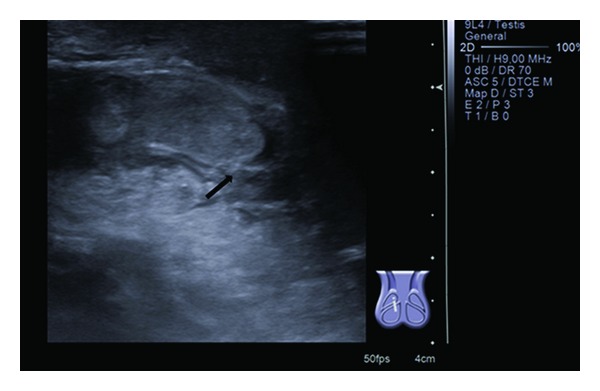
Scrotal ultrasound: scrotal inflammation—arrow.
